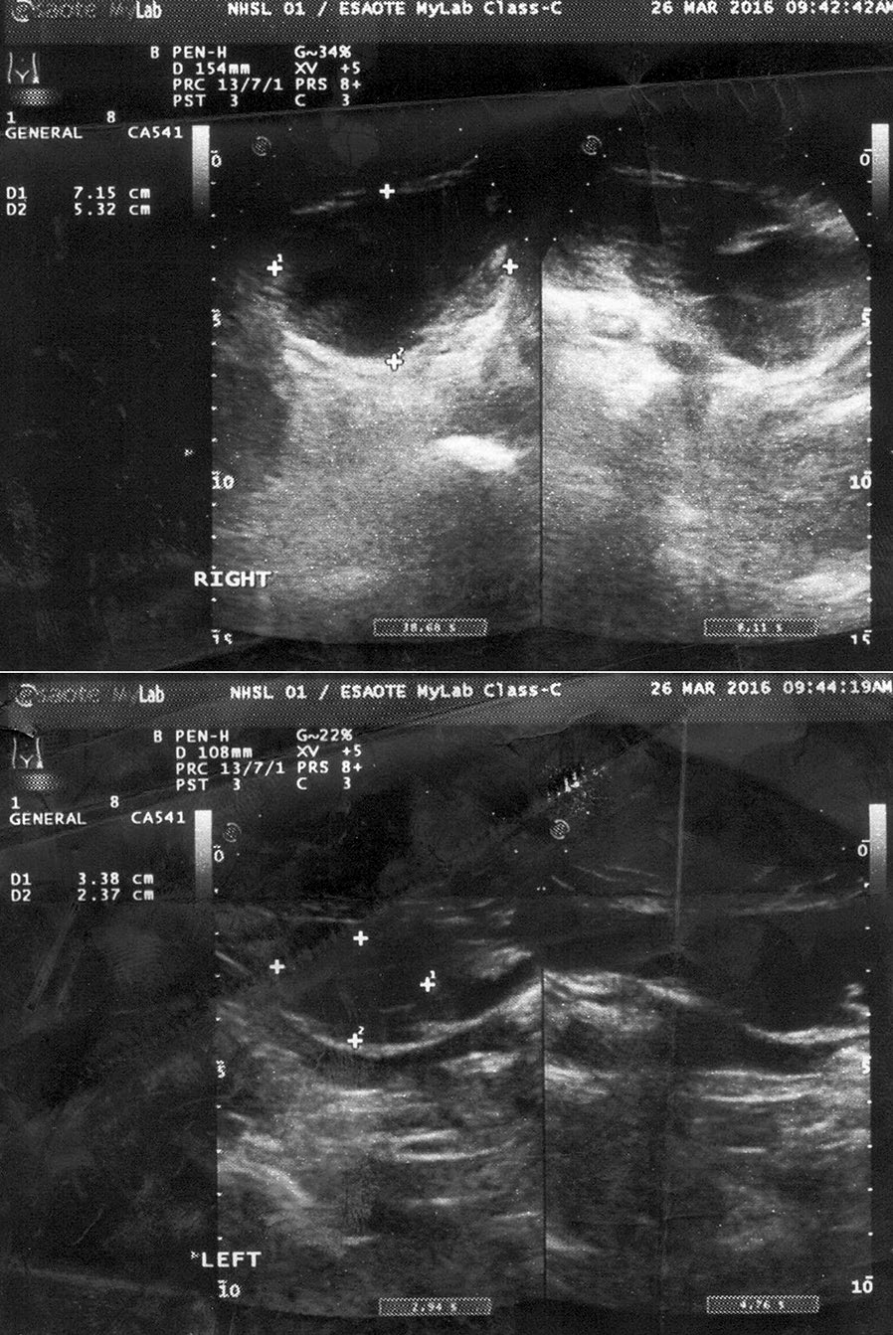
Image depicts the ultrasound findings which reveal a right sided hypo-echoic lesion (*upper image*) within the rectus sheath measuring 71 mm by 53 mm (depicted by cross hairs) and similar lesion within the left side rectus (*lower image*) sheath measuring 33 mm by 23 mm (depicted by cross hairs)
